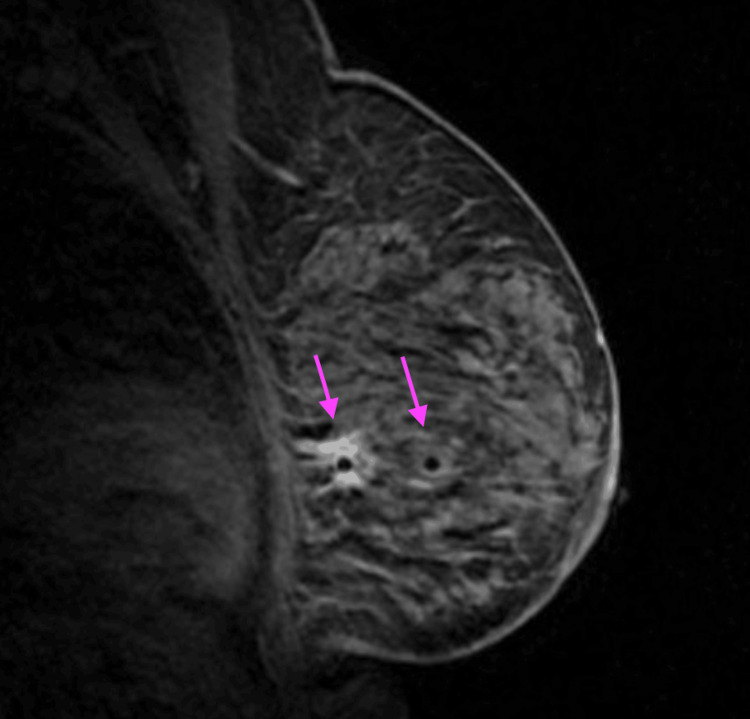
MRI-guided biopsy of the enhancing mass in the left breast. T1-weighted post-contrast, non-fat-saturated sagittal MRI intra-biopsy image demonstrated the placement of a biopsy needle in both the middle and posterior aspects of the mass (arrows).
